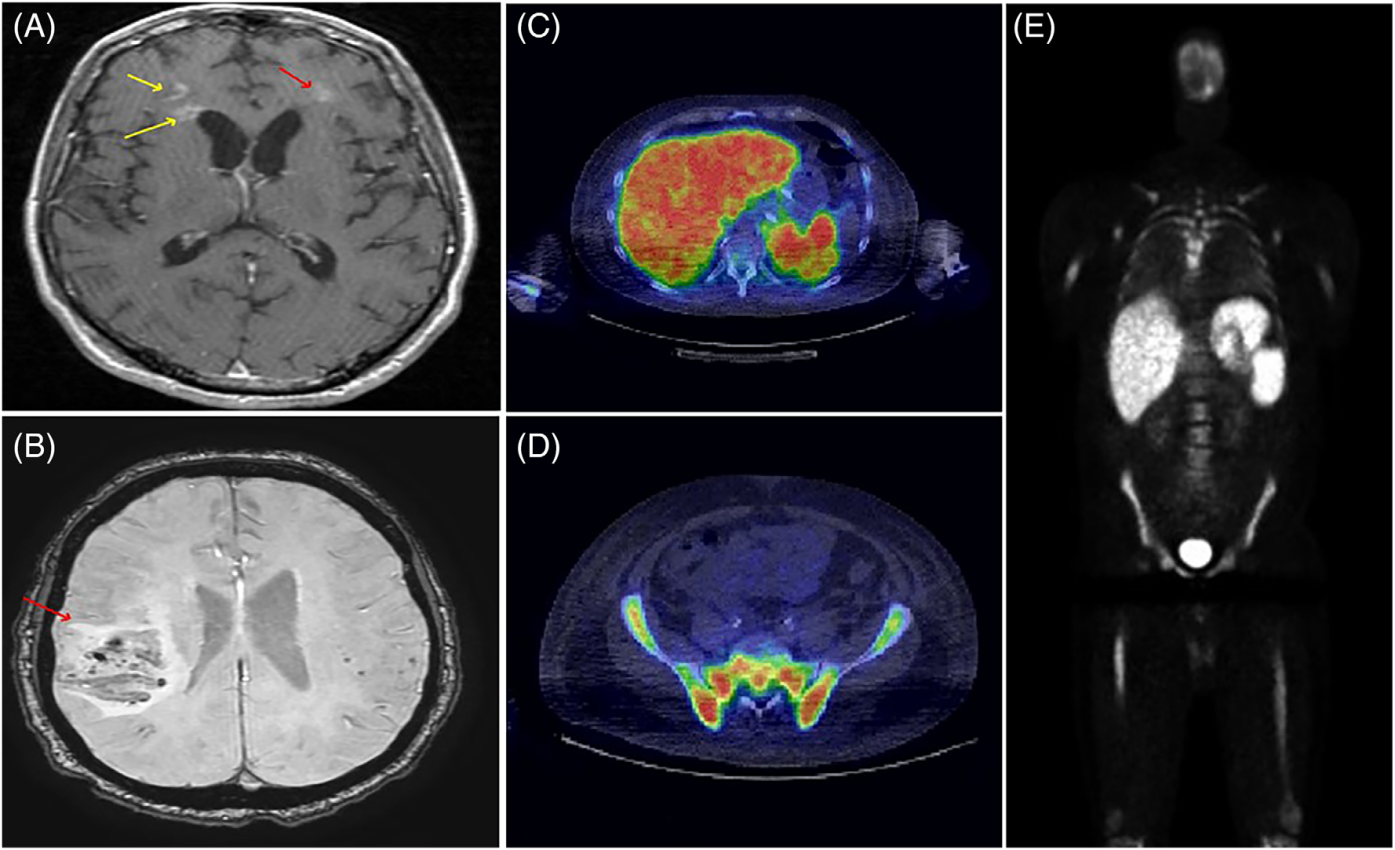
(A) Axial T1 MRI brain showing multifocal enhancing lesions in bilateral frontal lobes. (B) Axial SWI MRI brain showing mass lesion in the right parietal region with surrounding mass effect and compression of the right ventricle. (C–E) PET CT with increased FDG avidity in liver, spleen, and bone marrow with SUV 12.4 in bone marrow [Color figure can be viewed at wileyonlinelibrary.com]
